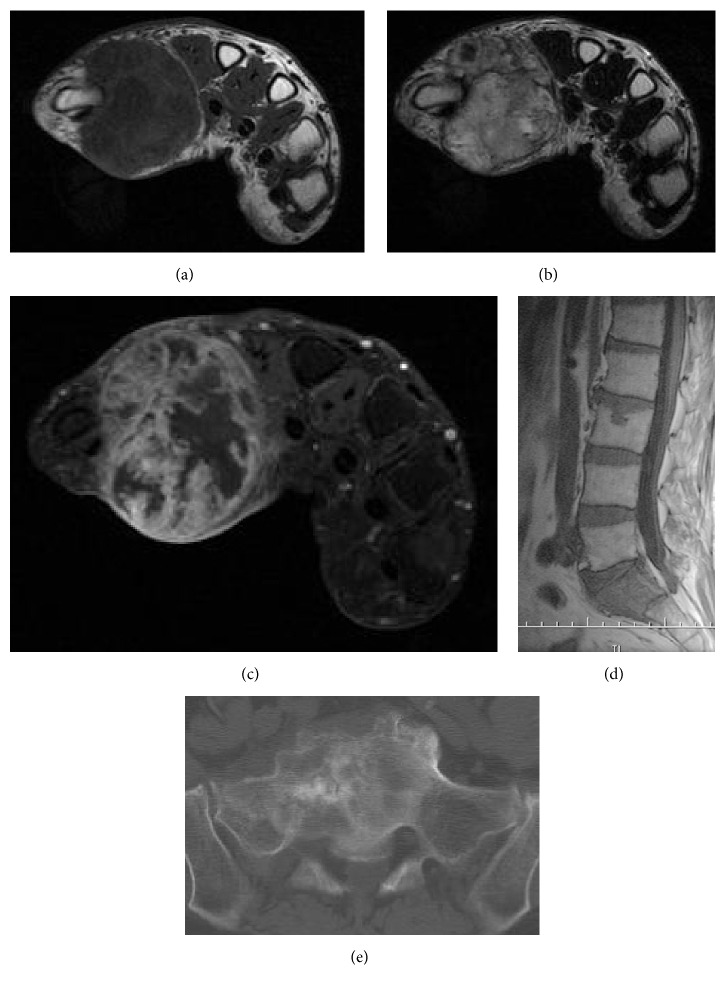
(a) T1 weighted, (b) T2 weighted, and (c) enhanced MRI images of the right hand suggested a malignant neoplasm. (d) MRI and (e) CT of the sacrum showed a lytic and sclerotic lesion, which was regarded as a prostate cancer metastasis.
